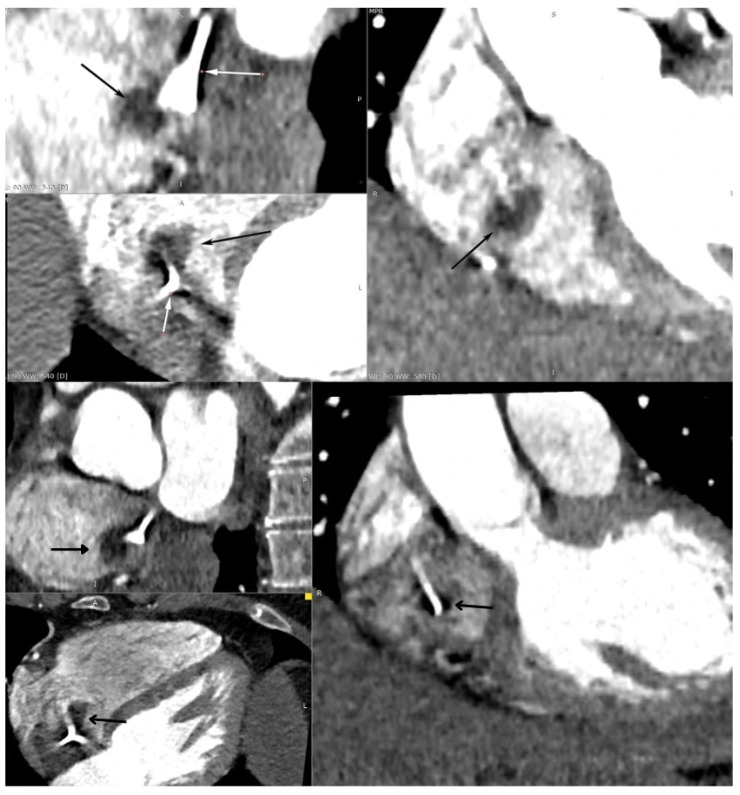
Subsequently, the patient underwent computed tomography angiography, which revealed a well-defined, oval structure measuring 15 × 20 × 18 mm located in the RA, just beneath the tip of the indwelling vascular access port. Given its proximity to the catheter tip, the possibility of an intracardiac thrombus could not be definitively excluded. White arrow—intravascular port; black arrow—RA mass. The features from CT suggesting benign character included the small size (<50 mm) and it being a single mass and well defined, with no calcifications or contrast enhancement. The only feature indicating possible malignancy was the right heart location [1,2].
